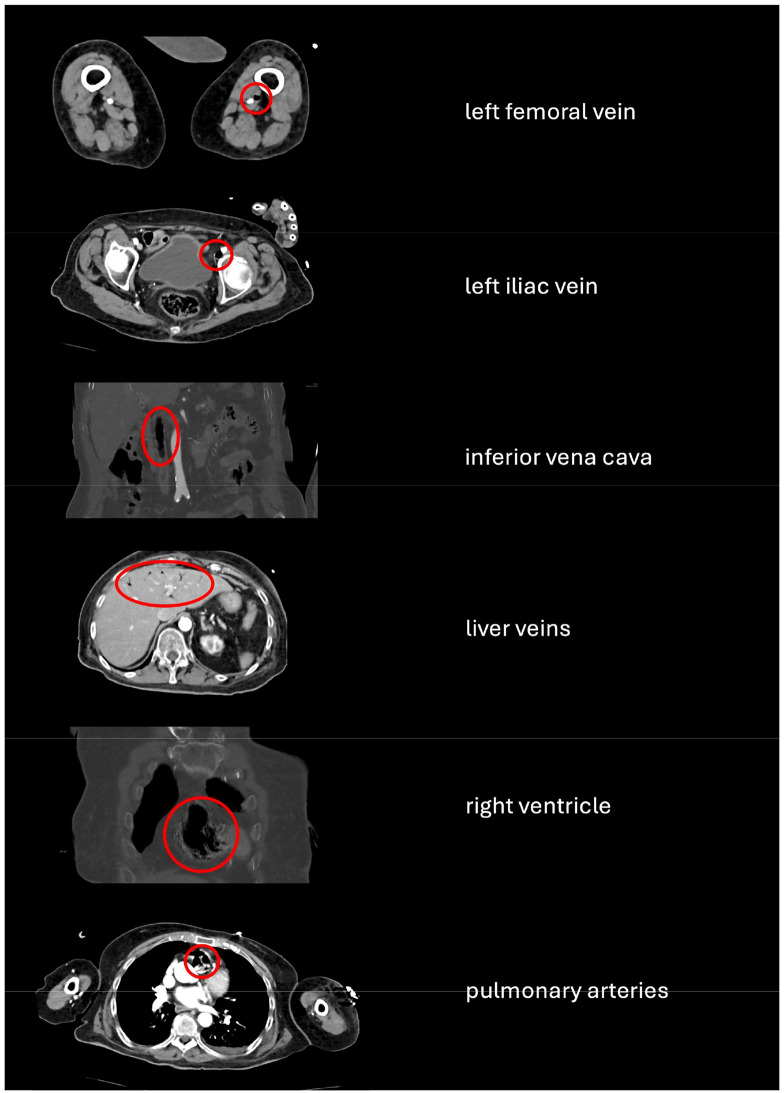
Contrast-enhanced computed tomography in the emergency room of the German armed Forces hospital in Ulm, showing air in various vessels. Axial view of the femoral, iliac and hepatic veins and the pulmonary arteries, coronary view of the right heart, sagittal view of the inferior vena cava. The red circles indicate intravascular air
